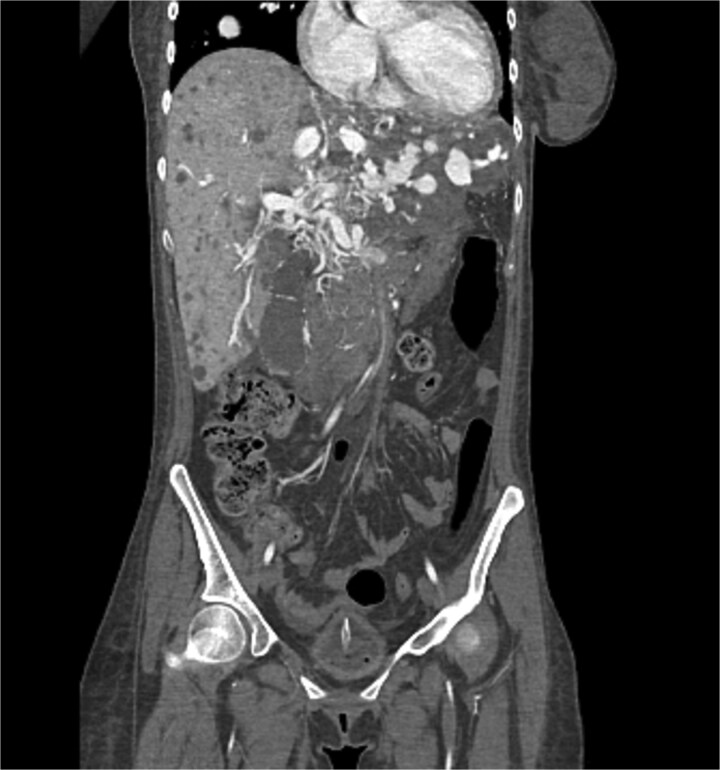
CT of the liver showed several AVMs and thrombosis.
